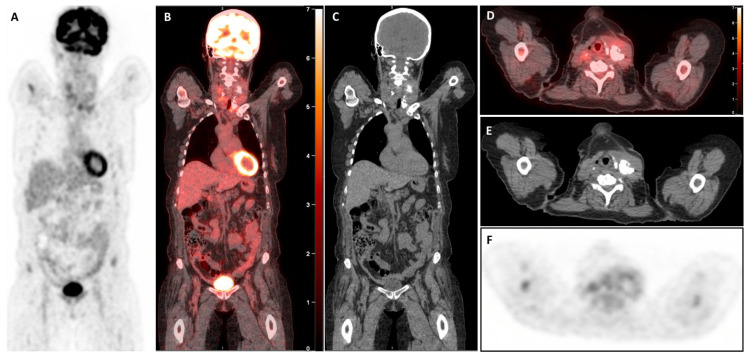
Interim [^18^F]FDG-PET/CT (iPET) images after two cycles of chemotherapy. The whole-body MIP (A) image shows a normal distribution of tracer throughout the body, associated with diffuse axial and appendicular skeletal activity of reactive nature, and a substantial absence of significant tracer activity in the neck. Coronal (B,C) and axial (D–F) unenhanced CT images of the neck reveal significant reduction in the size of the thyroid gland with calcifications in the left thyroid lobe (craniocaudal dimension from 14 cm to 10 cm). Subsequent [^18^F]FDG-PET/CT images show diffuse [^18^F]-FDG uptake in the residual thyroidal mass (SUVmax 3.0) less than hepatic uptake (SUVmax 3.1), suggestive of a complete metabolic response (Deauville score of 3).
